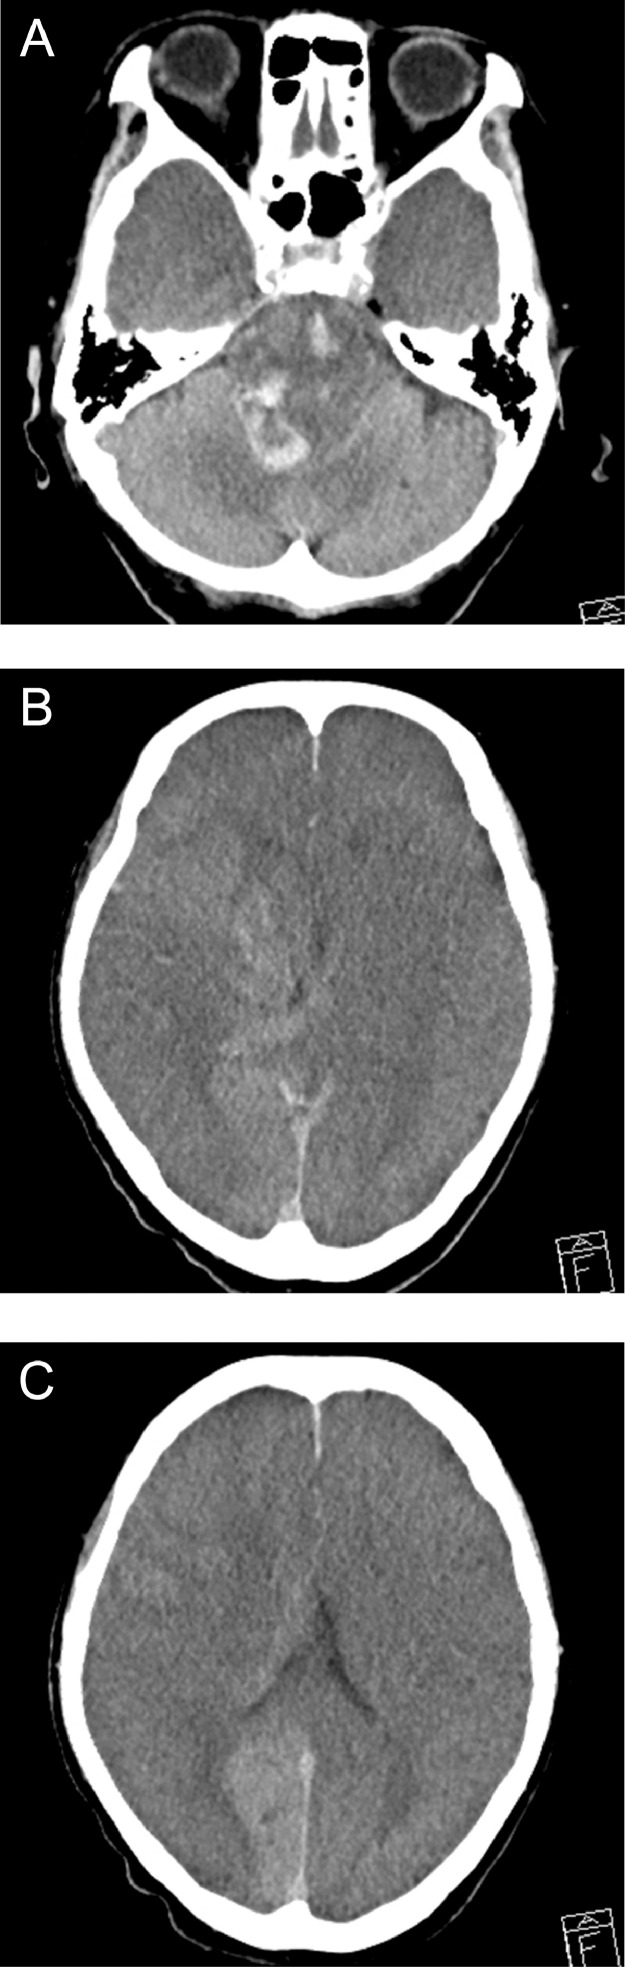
Computed tomography scan obtained on the day after onset demonstrating massive cerebral infarcts and marked brain swelling.
